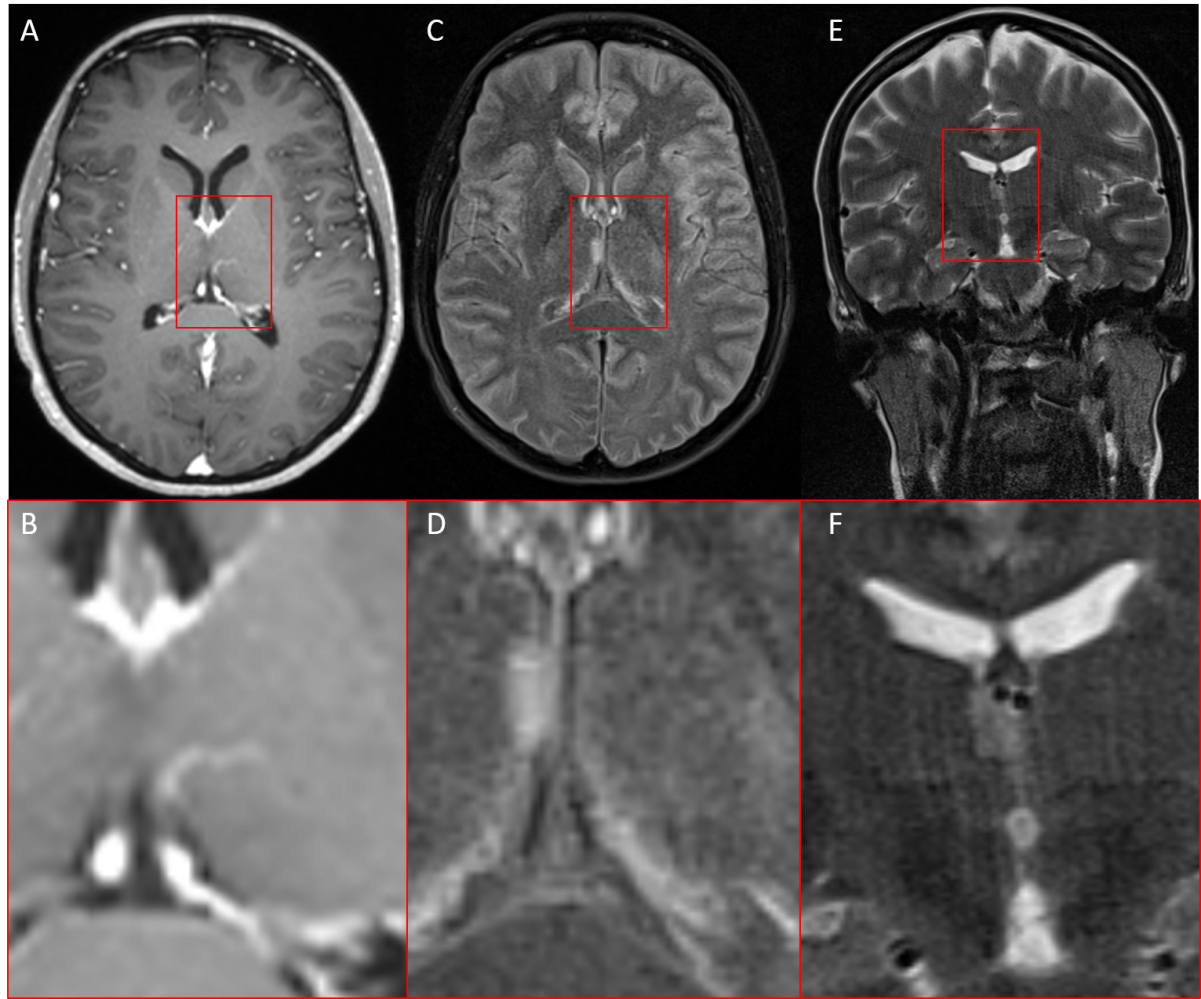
MRI of the same patient six years earlier. (A, B) T1w with contrast agent, (C, D) FLAIR (fluid-attenuated inversion recovery), (E, F) T2w coronar, In retrospect, a small FLAIR-/T2w-hyperintense lesion without contrast enhancement in the right thalamus adjacent to the third ventricle.
